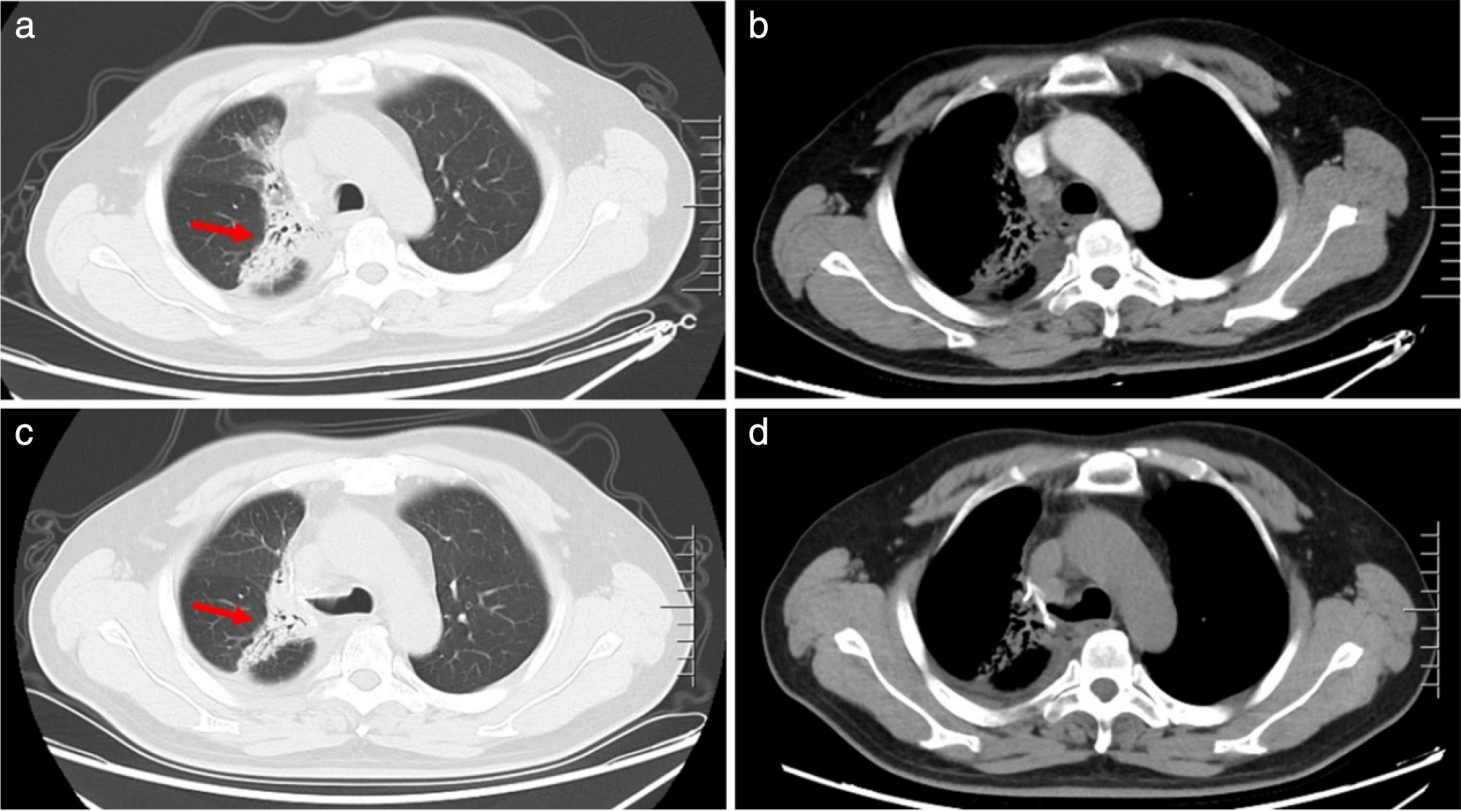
The patient presented with cough and chest pain on December 29, 2020. The CT images (a and b) revealed a radiation pneumonitis, improved after administration of anti‐inflammatory medications (c and d)
